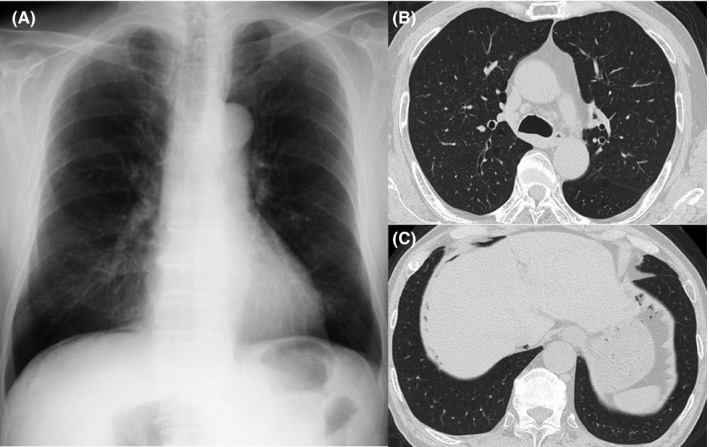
Radiological examination of Case 1. A, Chest X‐ray showed free air beneath the right diaphragm. B, C, Computed tomography showed mild pulmonary emphysema and intra‐abdominal air around the liver surface
